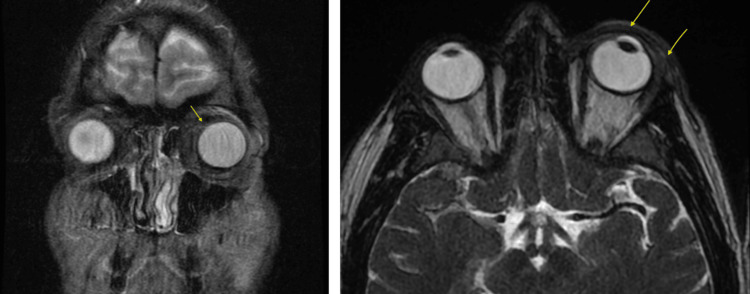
Coronal and axial MRI views of the orbits; the arrows denote periorbital edema and soft tissue swelling.
